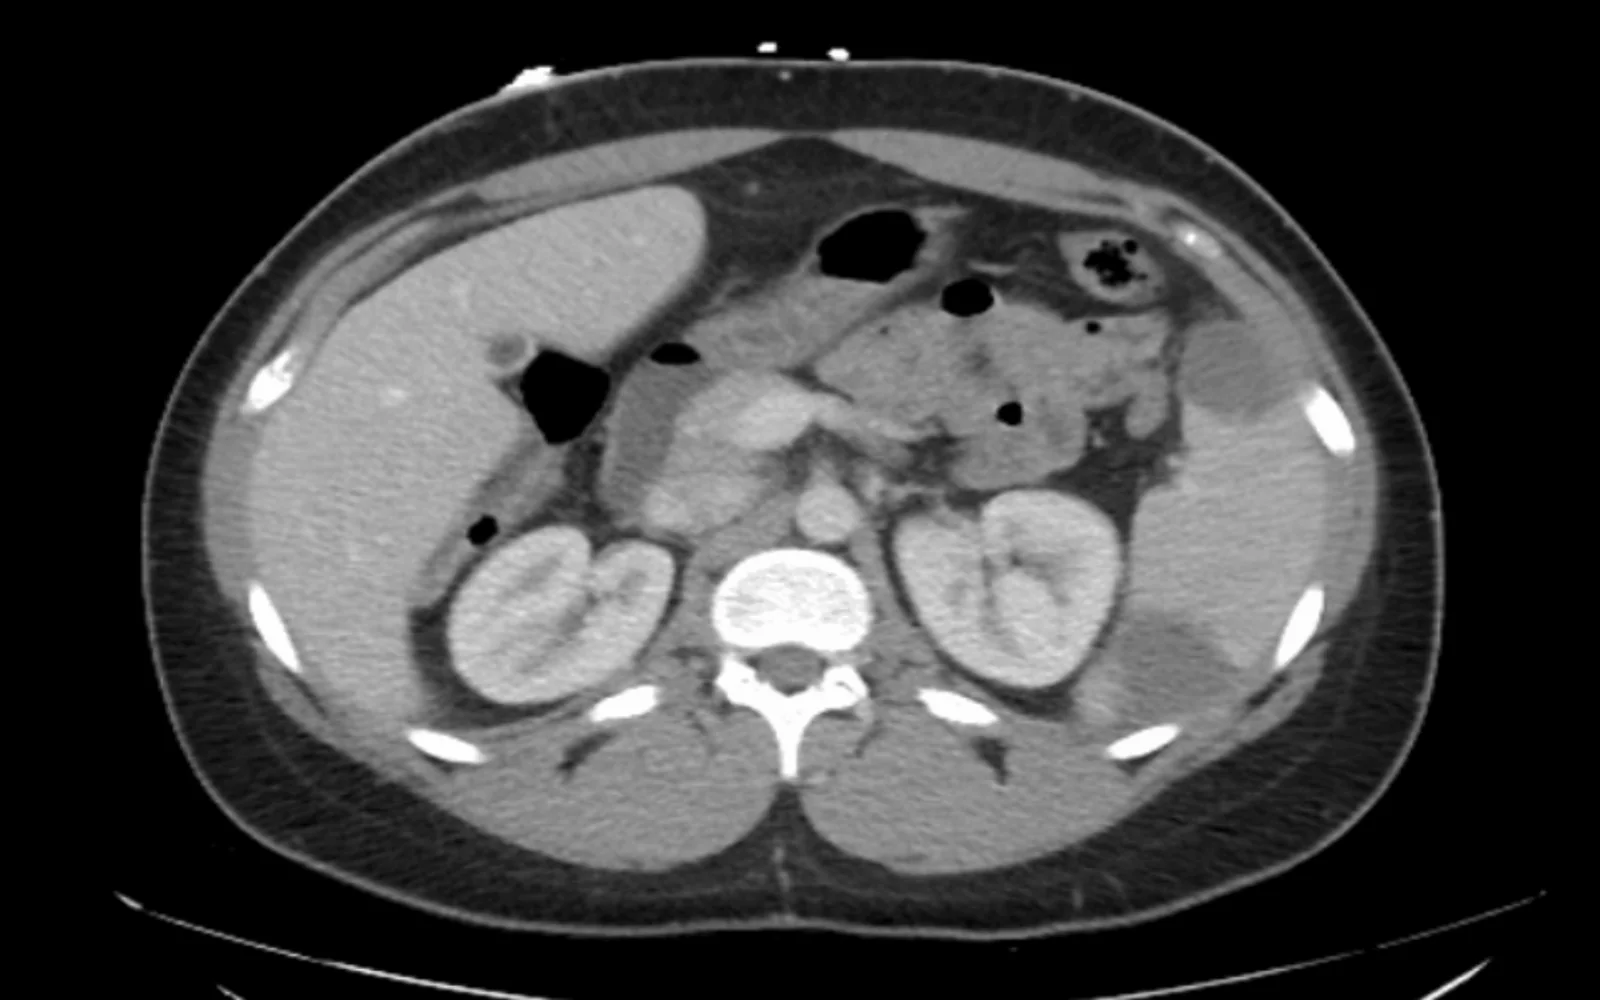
Contrast-enhanced computed tomography of the abdomen demonstrating splenomegaly with multiple peripheral wedge-shaped hypodensities consistent with splenic infarcts.
